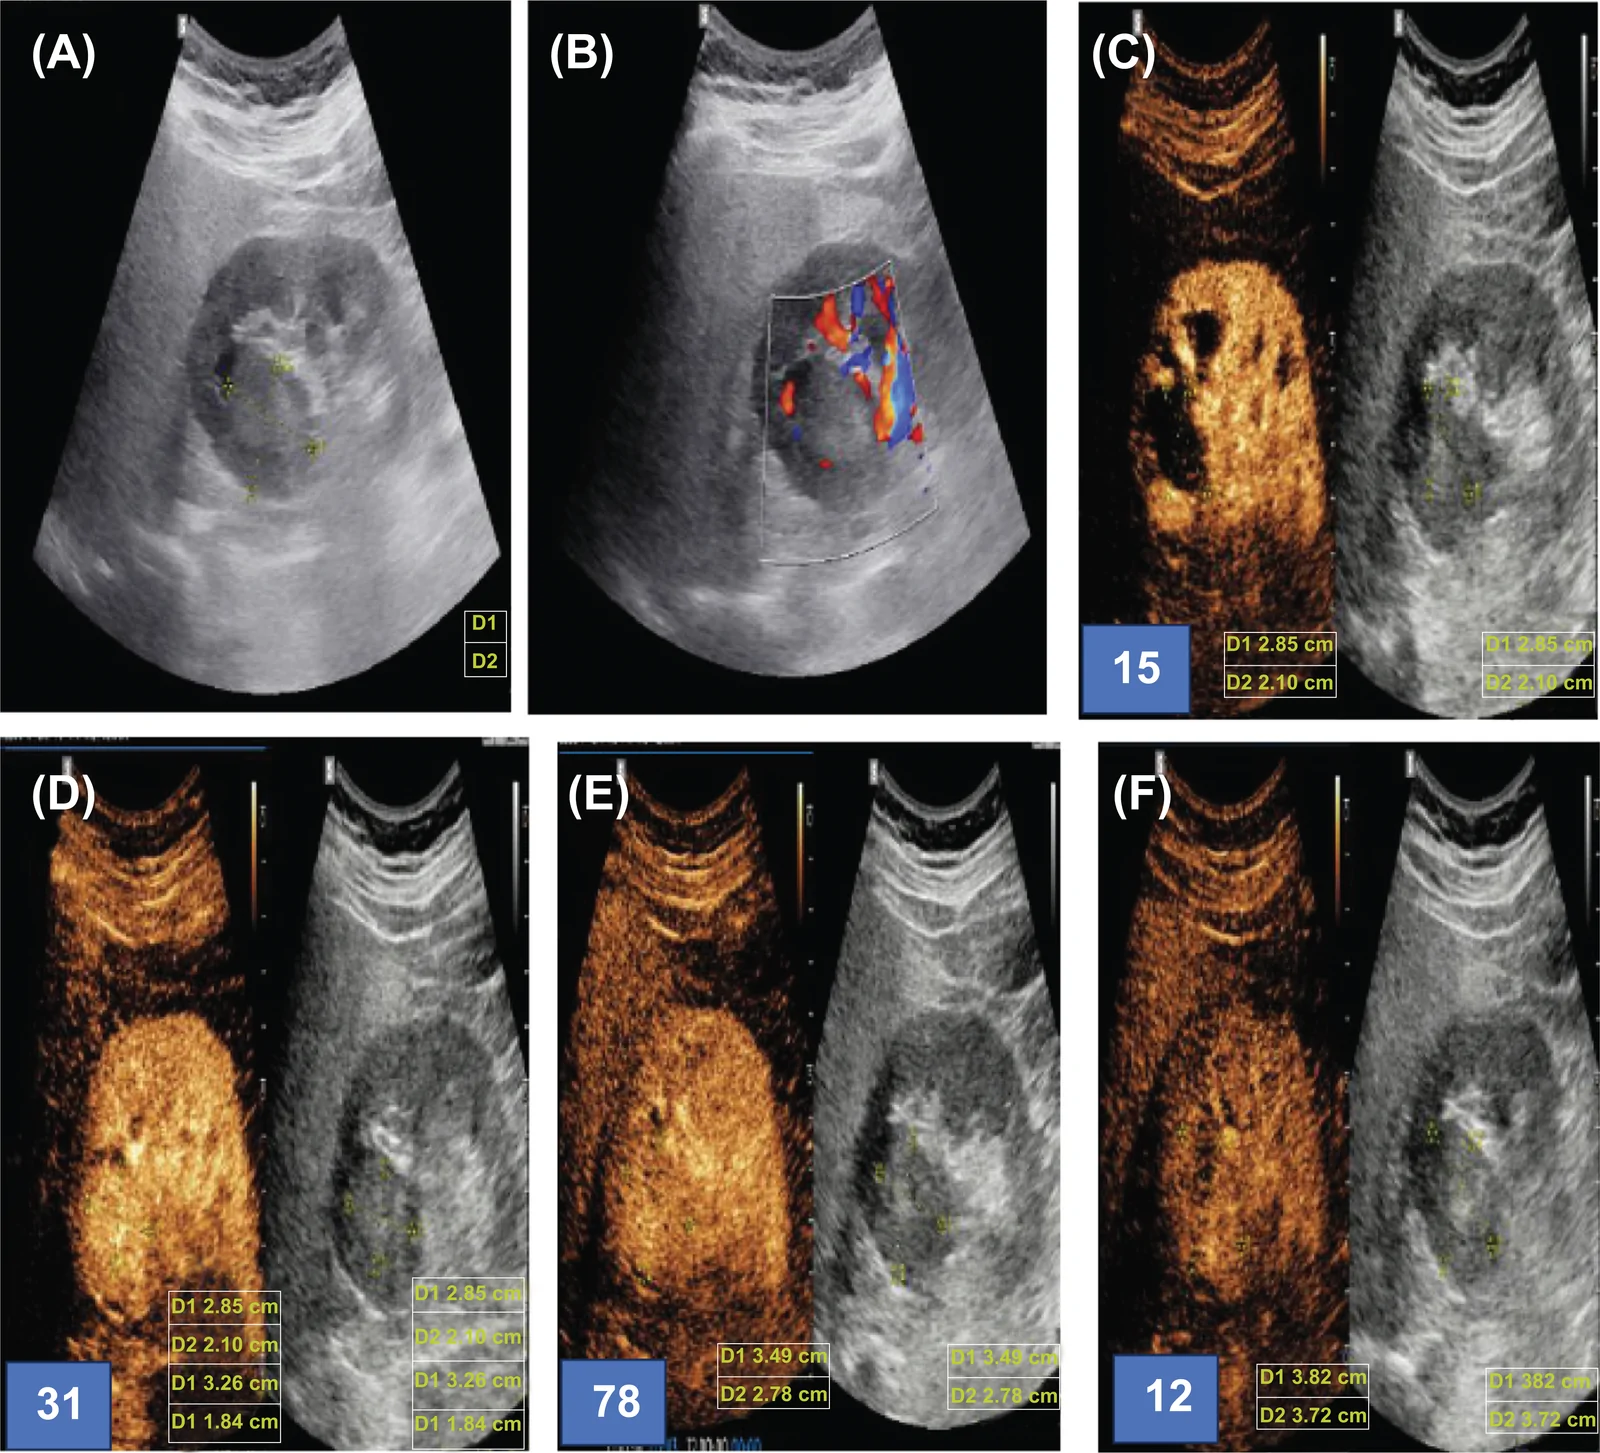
Patient Xu, a 28-year-old male. A: Two-dimensional ultrasound shows a lesion ultrasound examination which revealed a lesion situated within the renal pelvis of the right kidney. The lesion measures approximately 3.8 × 2.8 cm, exhibits an oval shape, and possesses relatively well-defined margins. No significant hydronephrosis was observed in the collecting system of the right kidney. B: Color Doppler Flow Imaging (CDFI) demonstrated a star-like blood flow signal surrounding the lesion, with sparse blood flow within the lesion itself. C: Upon administration of the SonoVue ultrasound contrast agent, the timing of enhancement was noted. At 15 seconds post-injection, the cortex of the right kidney was fully enhanced, whereas the lesion began to enhance at a slower rate. D: Between 18 and 31 seconds, the contrast agent gradually enhanced the lesion from its periphery to its interior. By 31 seconds, the lesion was completely filled with the contrast agent, achieving an enhancement level nearly equivalent to that of the renal cortex. E: At 78 seconds, the contrast agent in the renal cortex began to dissipate slowly, at which point the lesion appeared slightly more enhanced than the surrounding renal parenchyma. F: At 120 seconds, the contrast agent in the renal cortex further fades, and the lesion in the right kidney fades slightly later than the surrounding renal parenchyma.
